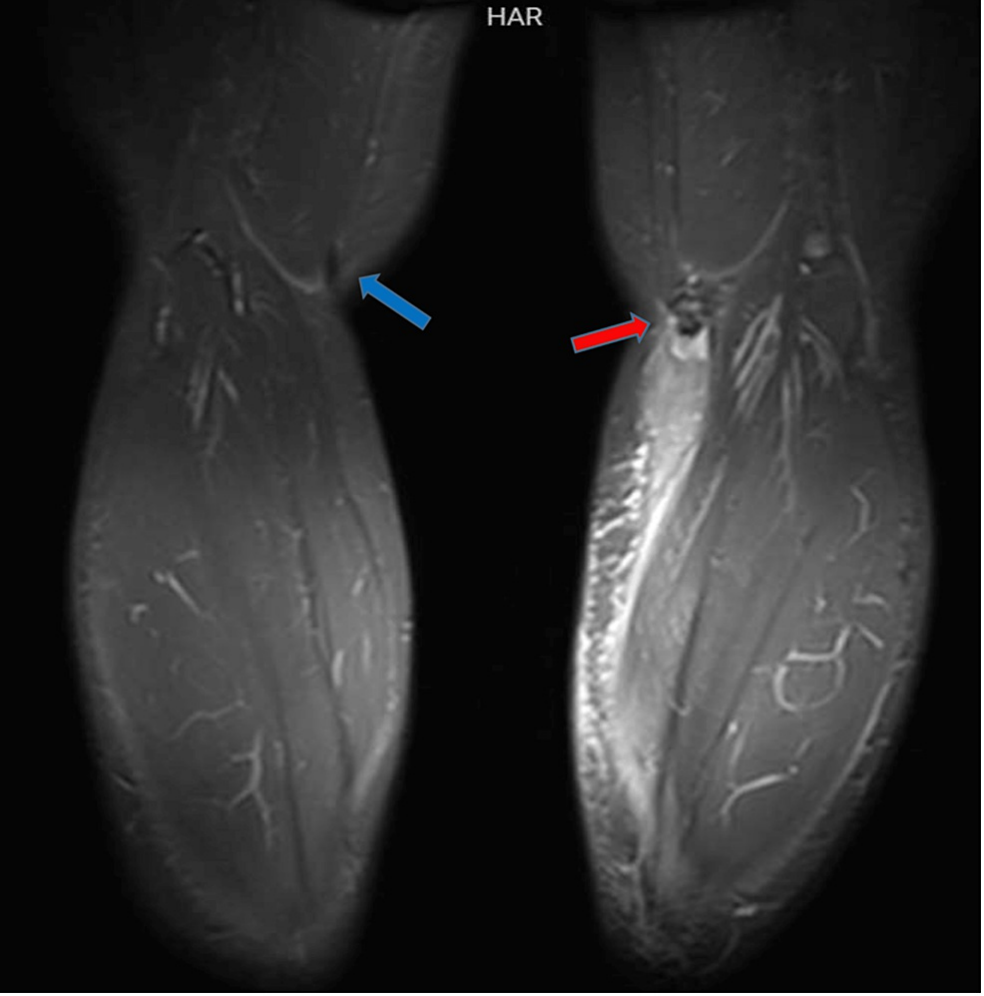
Coronal short-T1 inversion recovery magnetic resonance imaging shows retraction of the ruptured left semitendinosus tendon (red arrow) with high signal fluid collection as well as overlying high signal subcutaneous edematous changes. Note the normal appearance of the right semitendinosus distal tendon (blue arrow).
